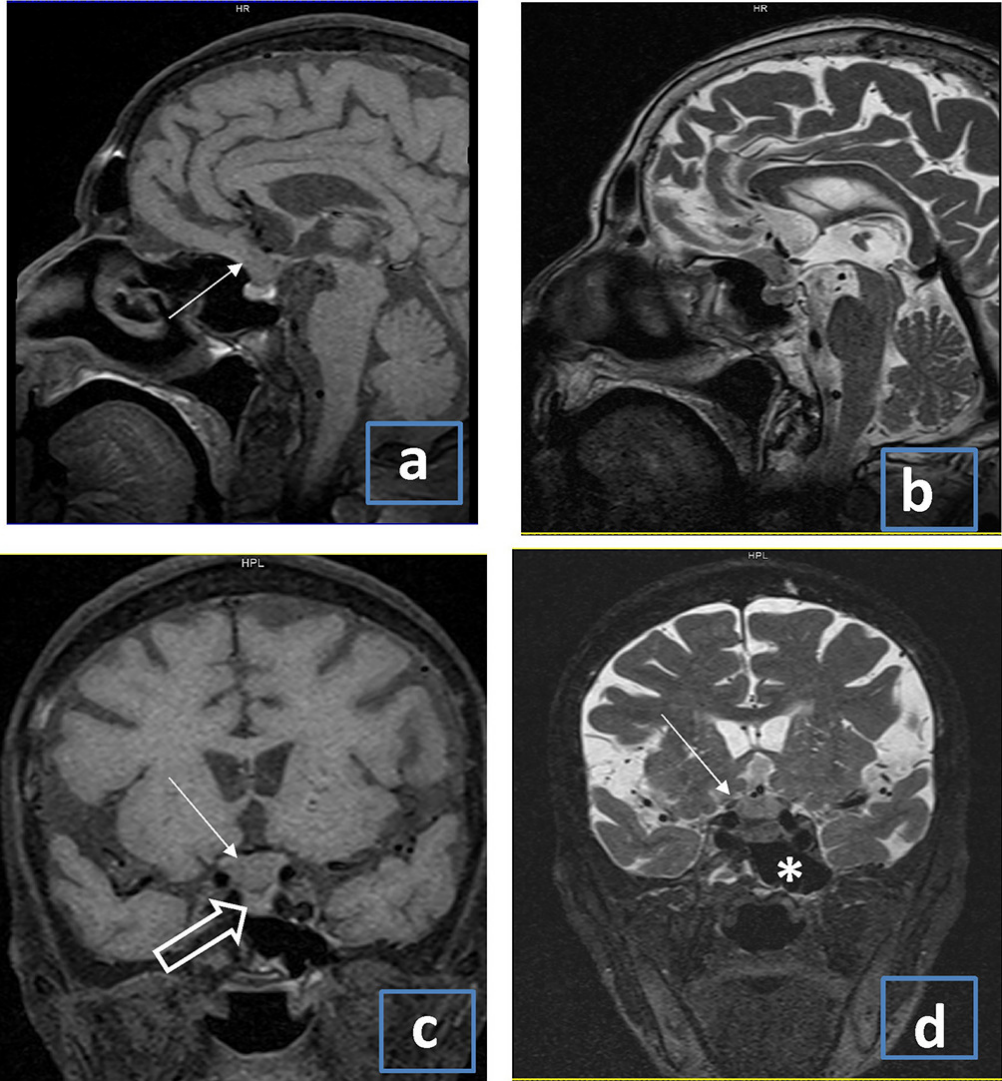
(a–d) MRI of the brain. *T*
